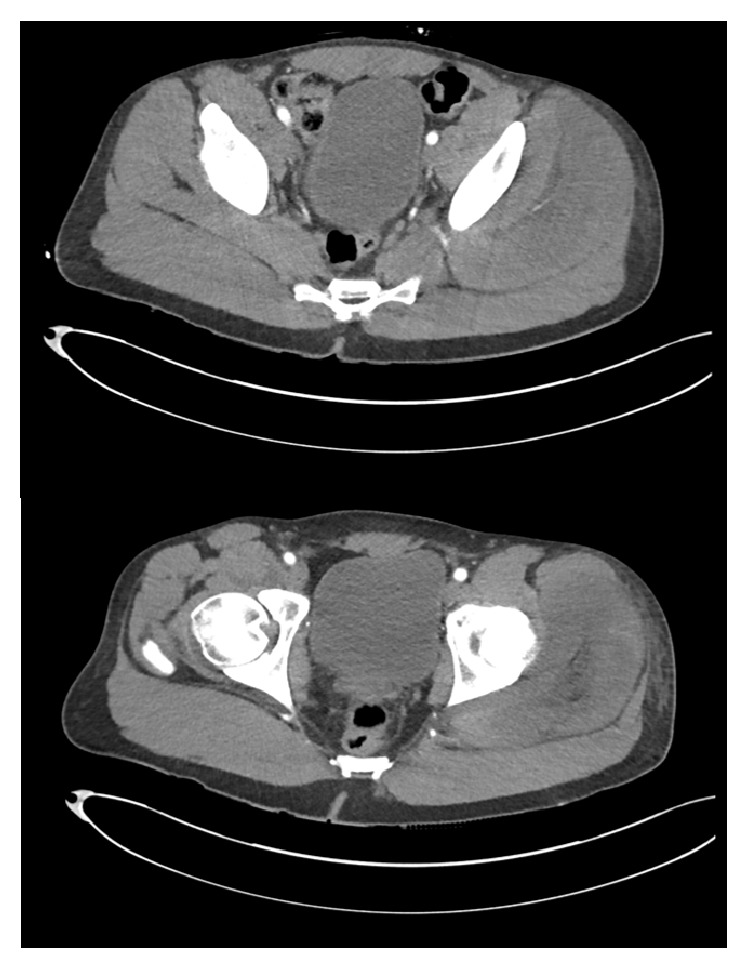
CTA of the gluteal vasculature showing intact vasculature with obvious swelling of the gluteal muscles within the left gluteal compartment, compared with the right.
